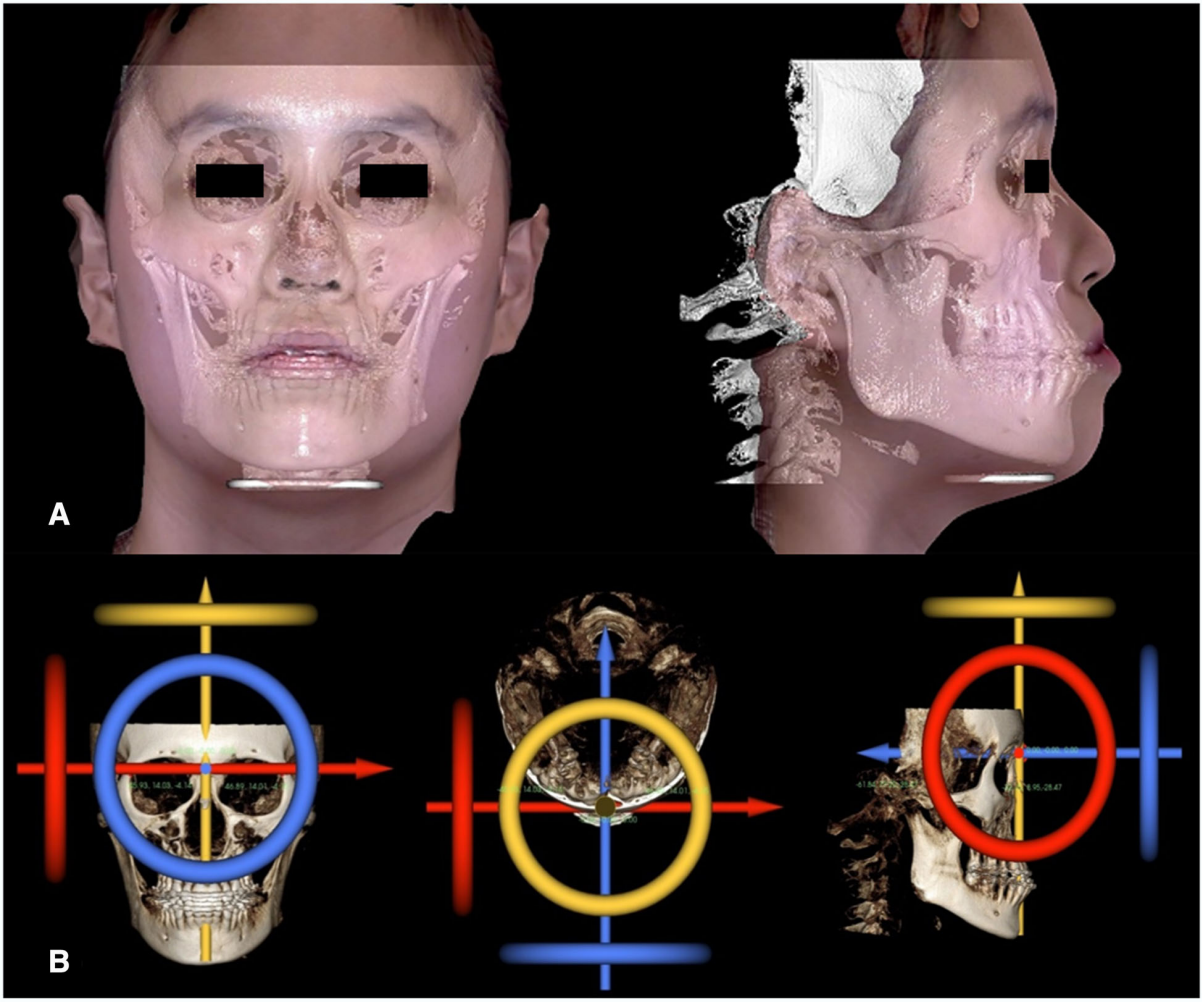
Superimposed 3D image of CBCT and facial scan (a) and 3-dimensional coordinate system (b)
